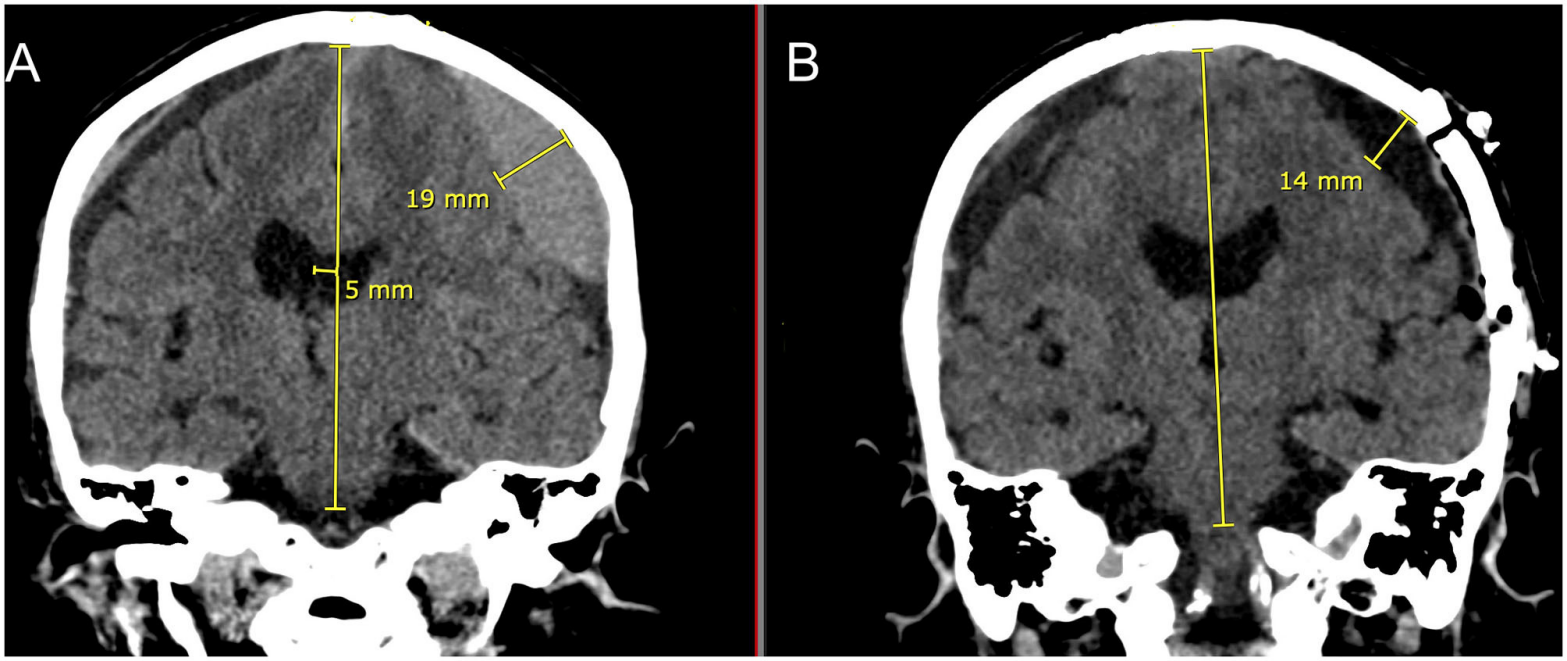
Eighty three yo F who suffered a ground level fall who was on aspirin. She had mild headaches and speech difficulties with a right upper extremity pronator drift. (A): Preoperative coronal CT scan demonstrating a left sided cSDH with midline shift. Note the lentiform shape afforded by the inner subdural membrane that is displacing the underlying cortex. There is a smaller right sided acute on chronic SDH. (B): Post-operative coronal CT scan done within 1–2 h of reaching the recovery room. Note the decrease in the mass effect and midline shift with near complete removal of the cSDH and re-expansion of the brain on the left. There is very little intracranial air. On the right sided there is increased prominence of the subdural space and the acute on chronic subdural hematoma.
